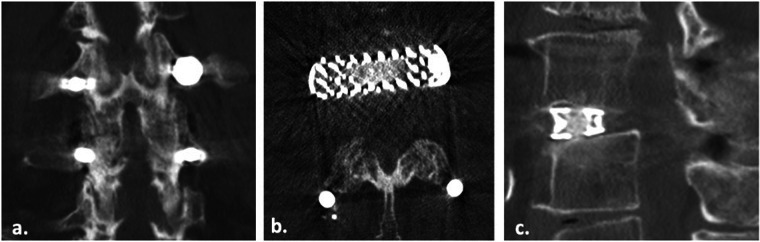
Coronal (a), axial (b) and sagittal (c) CT scan images of a 72 years old patient showing complete fusion of zygapophyseal facet (Pathria Grade III) and interbody bony bridge (BB) fusion.
